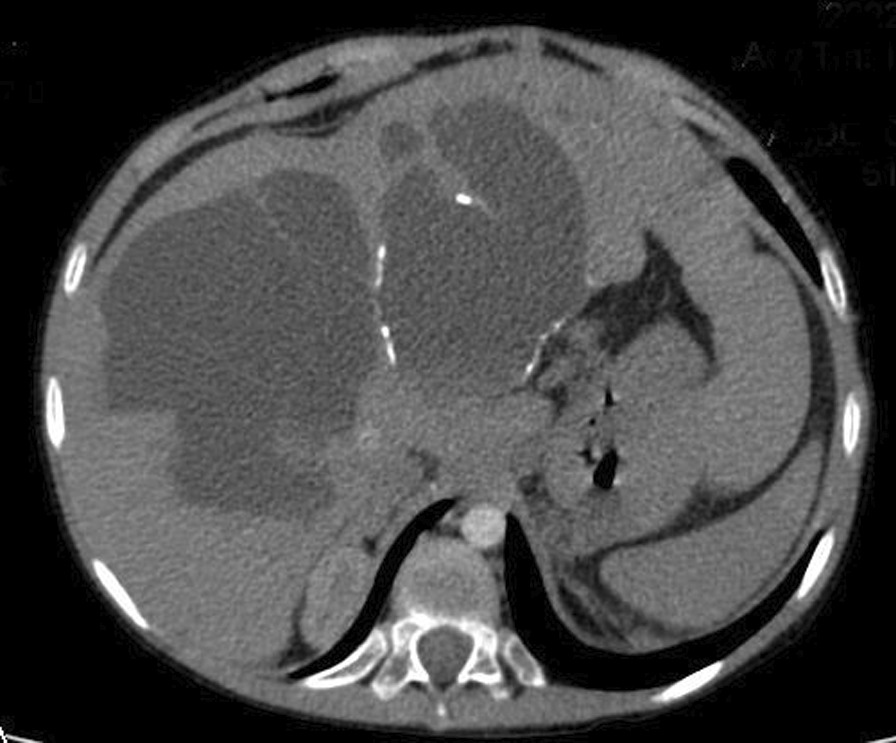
Abdominal CT scan with contrast showing intrahepatic cystic formations
